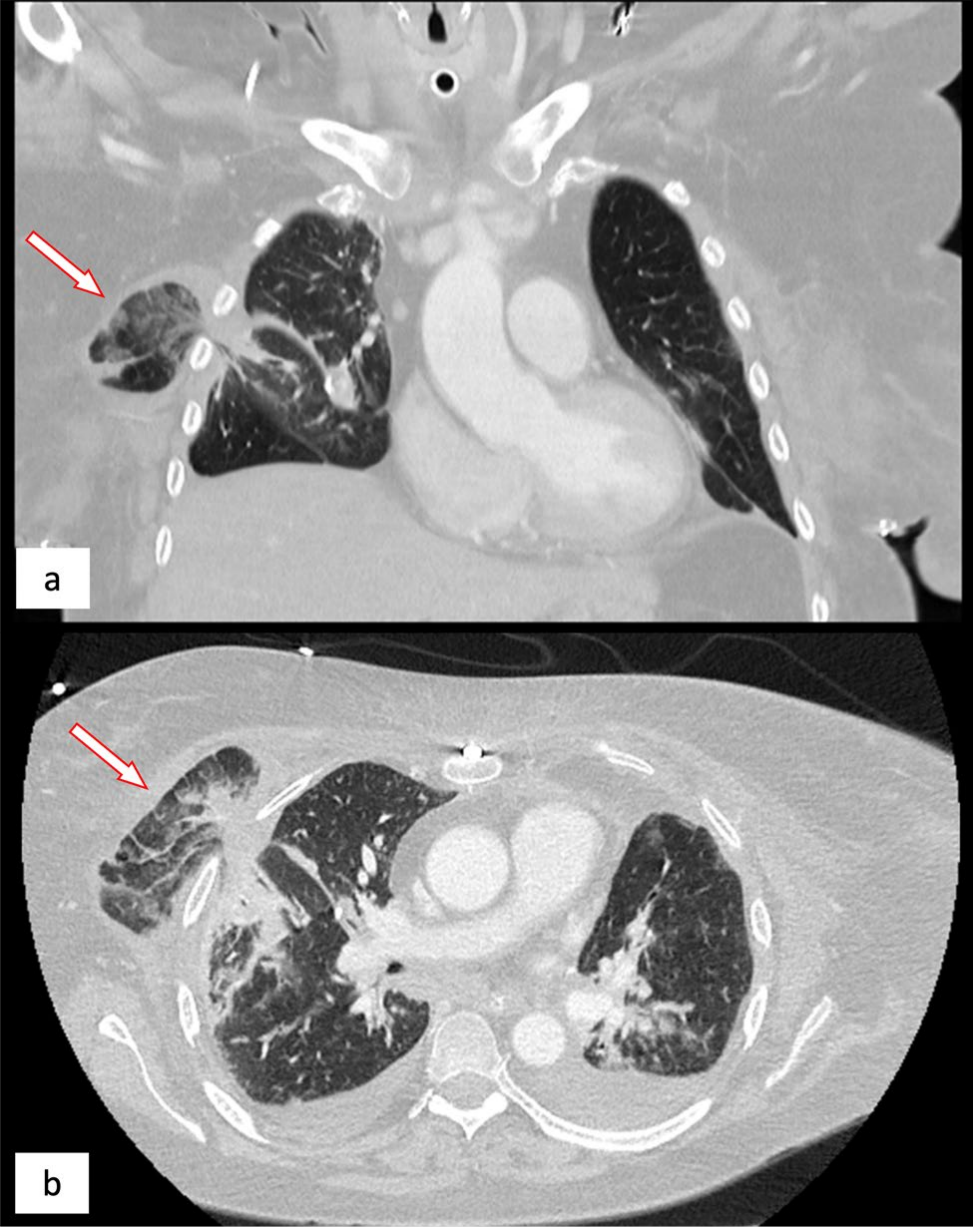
Chest CT scan, coronary (a) and transversal (b) axis. Arrows highlighting right lung herniation at 4th intercostal space
